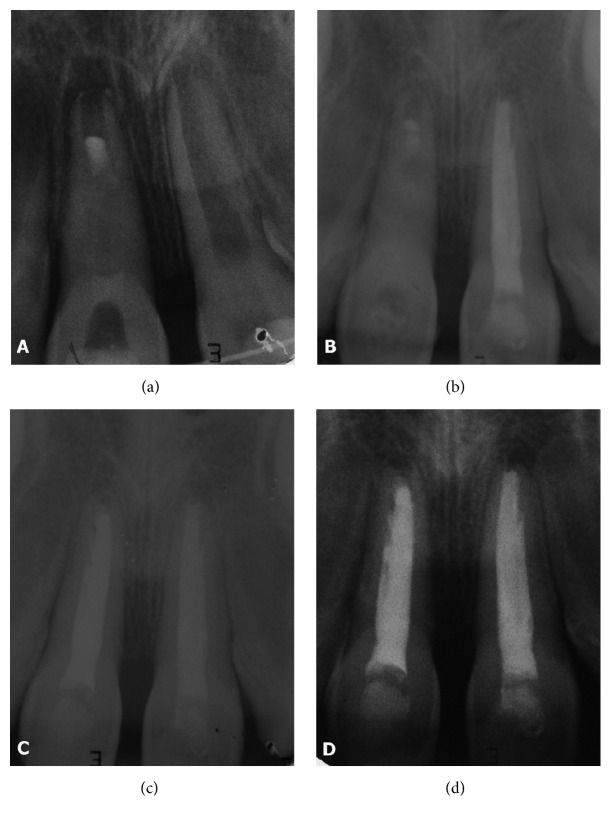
(a) Initial radiograph after tooth replantation (tooth 11), evidencing the incomplete root formation, the MTA apical plug about 2 mm inside of root canal, and the intracanal medication with calcium hydroxide-based paste. (b) Radiographic examination after 2 months, revealing apical closure and slight increase in root length of the replanted tooth (tooth 11). Endodontic treatment was performed on the left central incisor (tooth 21). (c) Radiographic examination 6 months after treatment of tooth 11, revealing apical closure. (d) Final radiographic examination, after a follow-up of 1 year and 6 months, revealing apical closure and no sign of external root resorption of the replanted right central incisor (tooth 11).
